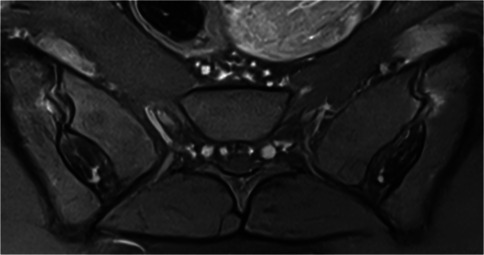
A 38-year-old woman with bilateral dysmorphic SIJ on axial oblique FS T2-weighted image: the central aspect of the joint is affected on the right side, the posterior aspect of the joint on the left side. Both are associated with subchondral edematous changes
